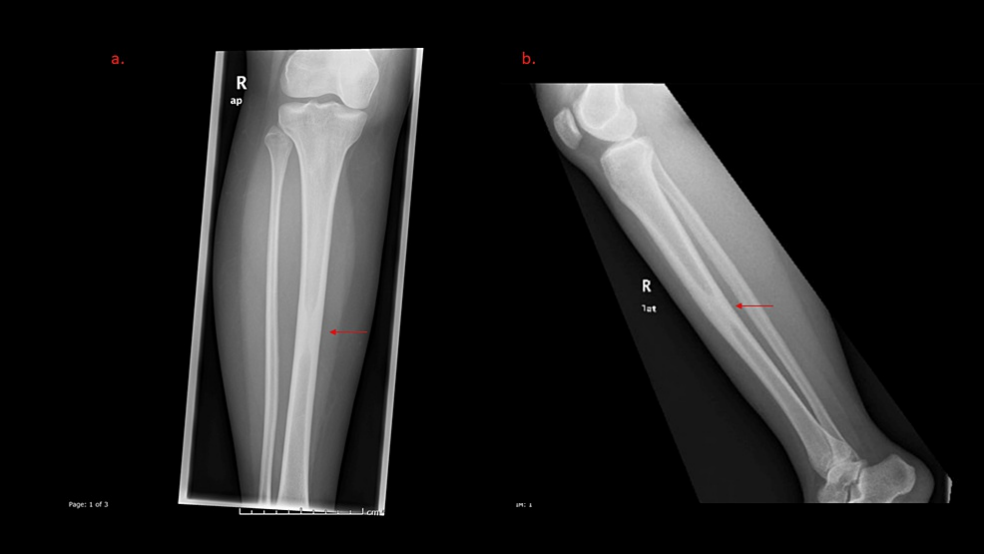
Frontal (a) and lateral (b) radiographs of the proximal tibia and fibula. Focal circumferential endosteal/intramedullary thickening and sclerosis involving the middle shaft of the tibia (arrows). It shows no destructive pattern, periosteal reaction, or soft tissue component.
